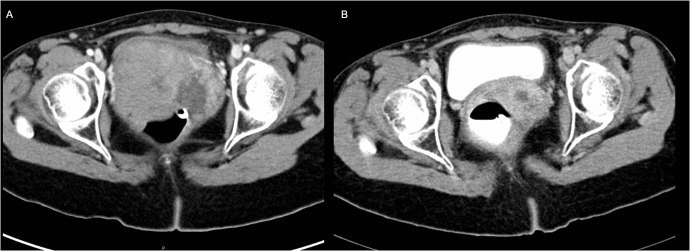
Radiographic images of Case 1. Pelvic computerized tomography (CT) scan: (A) Left image at time of presentation that shows a large hetergenous pelvic mass (9×8.8×5.5 cm) involving the vagina and upper part of the cervix. (B) Right image is taken post-radiotherapy and shows significant interval regression of the mass size.
